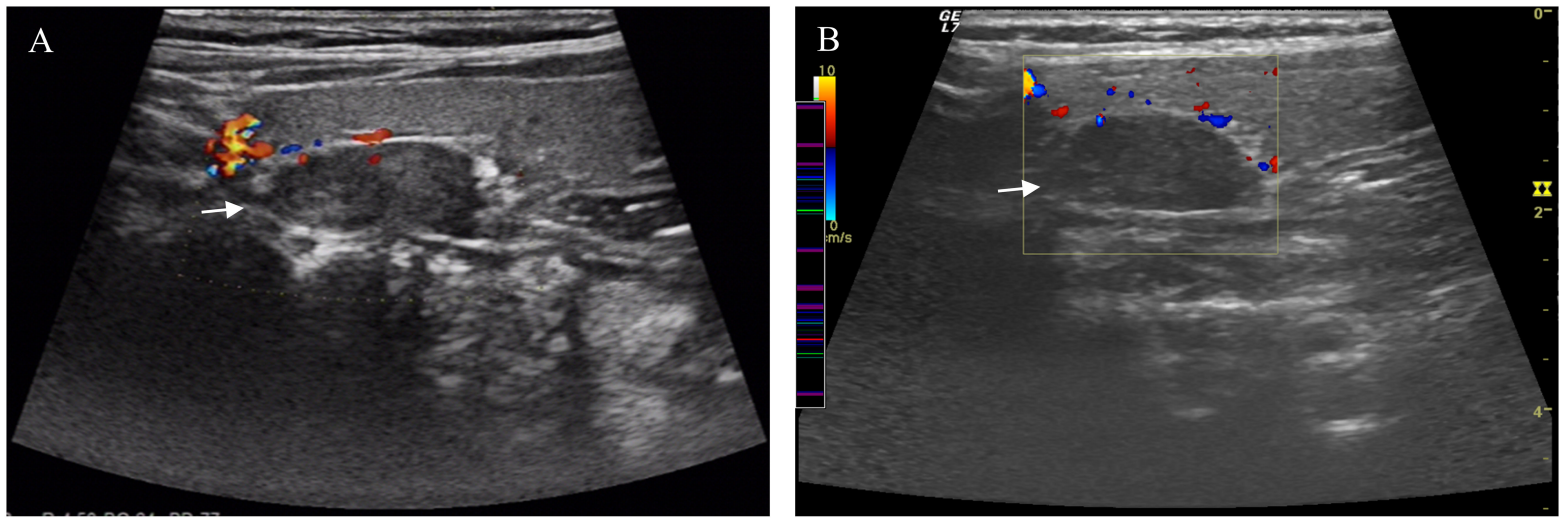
Ultrasound Imaging of the Parathyroid Glands. A hypoechoic nodule was observed on the left side of the parathyroid gland in the 2014 ultrasound (A). The same nodule showed no significant changes in the 2021 ultrasound (B). Arrow pointing to parathyroid nodule.
